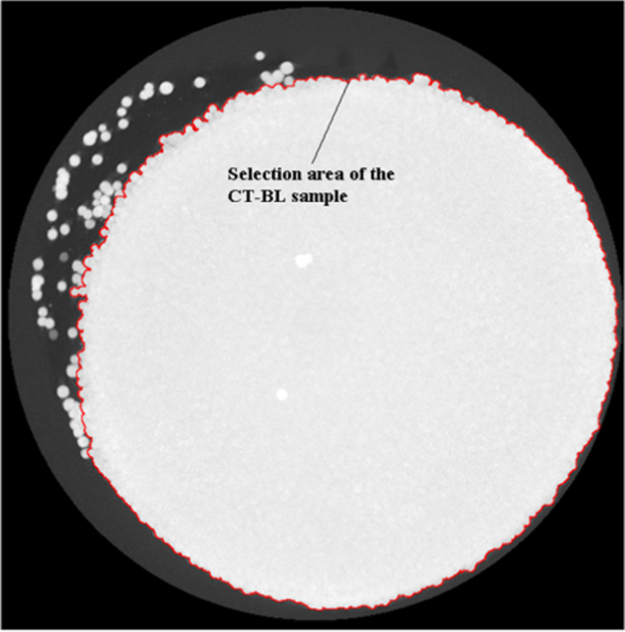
Maximum intensity projection image and resulting selection area (red boundary) of the CT-BL green part.
